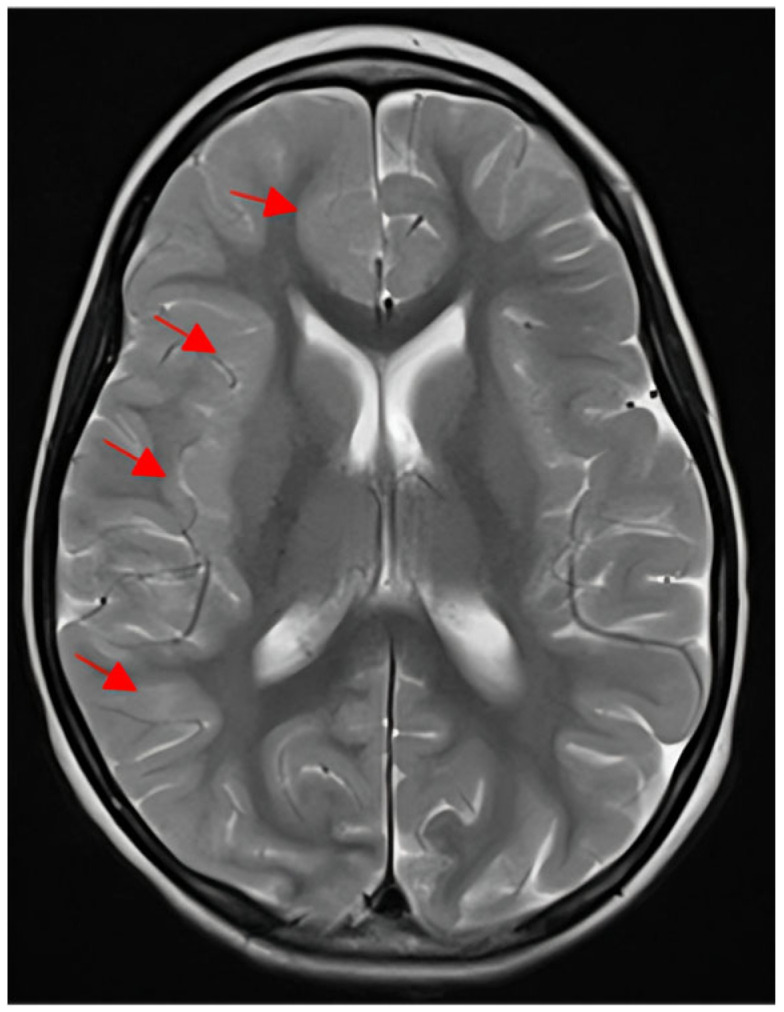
Brain MRI demonstrating bilateral myelination delay and right hemispheric cortical abnormalities (indicated with red arrows). MRI—magnetic resonance imaging.
